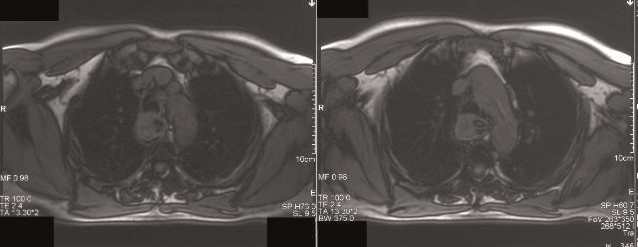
MRI T1-W axial image: a mass- like lesion is seen in the posterior mediastinum arising from the right anterior mucosal wall of the esophagus.
